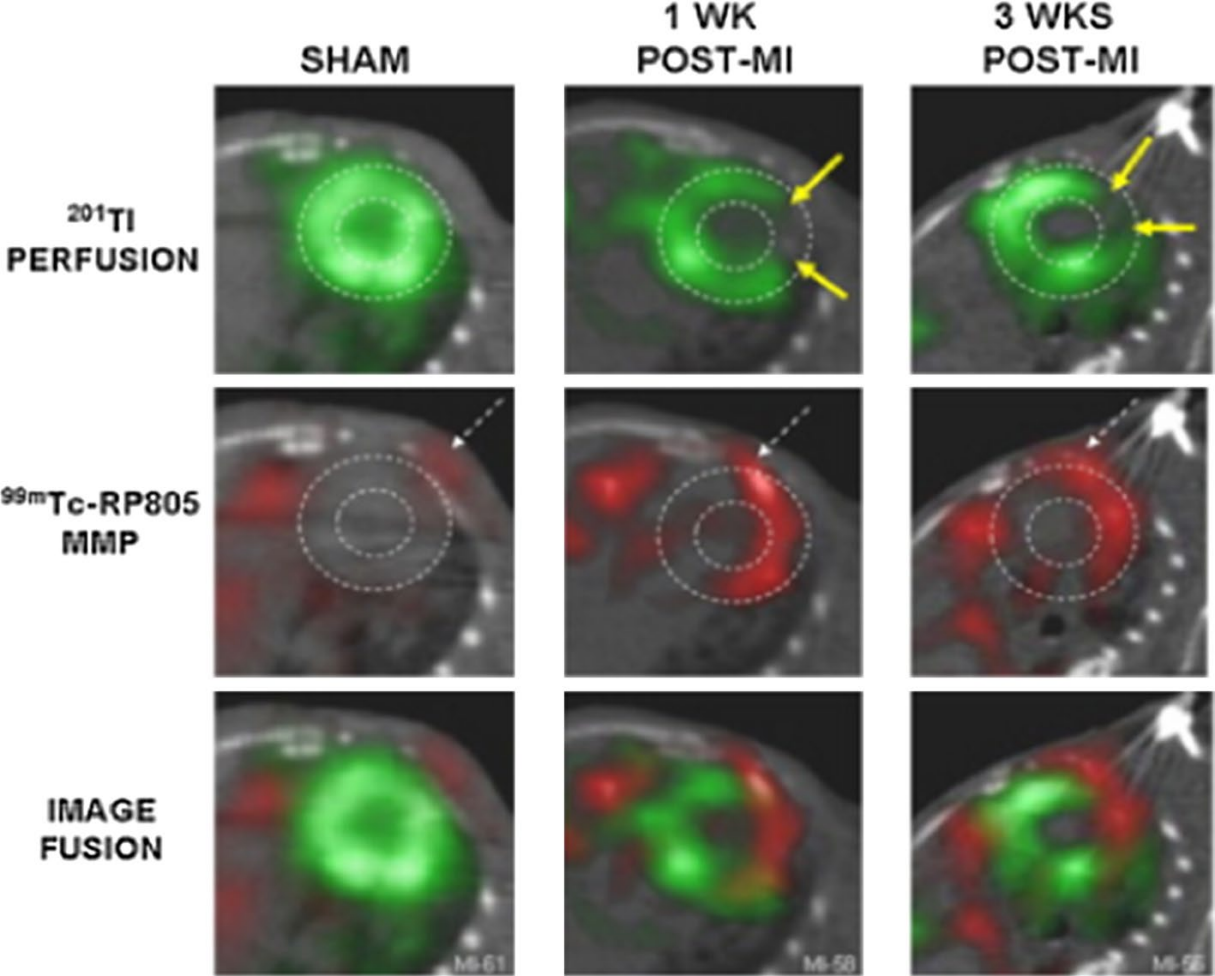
Thalium-201 perfusion imaging, Tc-99m-labelled MMP imaging, and fused images before (SHAM), 1 week and 3 week post-MI using SPECT/CT. Arrows show infarct zone, which is poorly perfused, where MMPs are detected [132]
